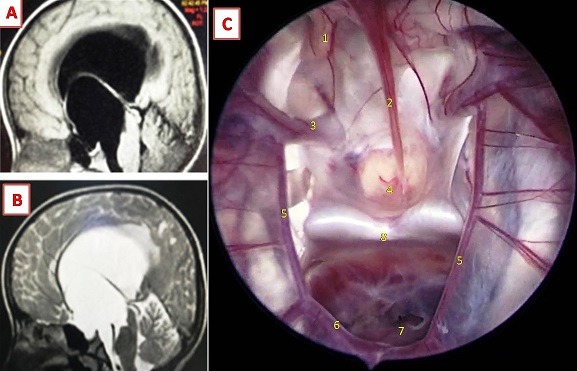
A) Sagital T1; B) T2-weighted MR image showing a large suprasellar arachnoid cyst with marked obstructive hydrocephalic changes; C) view of the suprasellar region during the endoscopic ventriculocystocisternostomy, showing the right optic nerve (1), pituitary stalk (2), internal carotid arteries (3), pituitary gland (4), posterior communicating arteries (5), posterior cerebral arteries (6), basilar artery (7), dorsum sellae (8)
